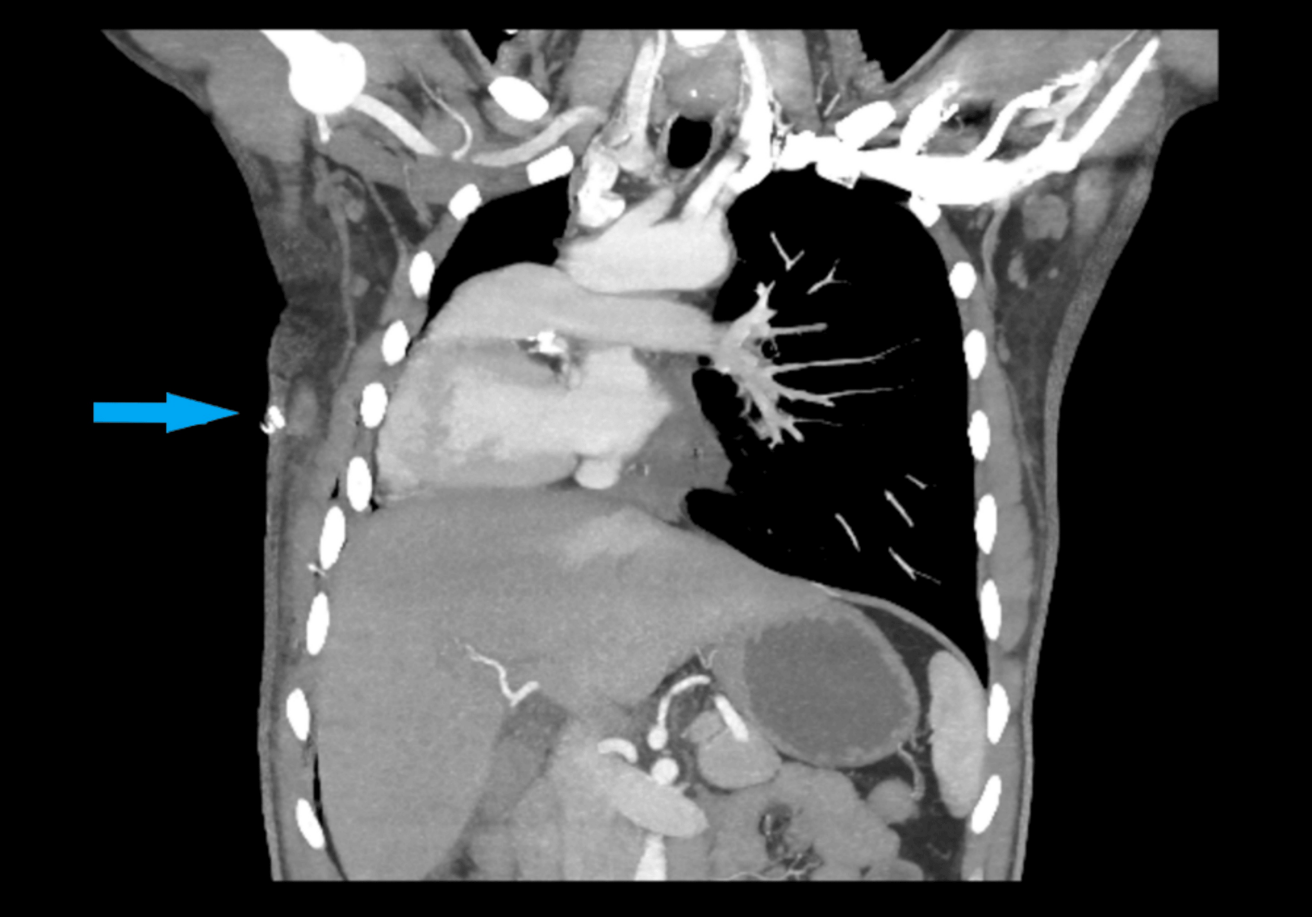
Coronal reformatted contrast-enhanced CT image of the thorax Coronal reformatted contrast-enhanced CT image of the thorax showing complete dextrocardia (blue arrow) with normal anatomical position of the aortic arch and great vessels. The pulmonary trunk is shown between the left ventricle and aortic arch. CT: computed tomography
